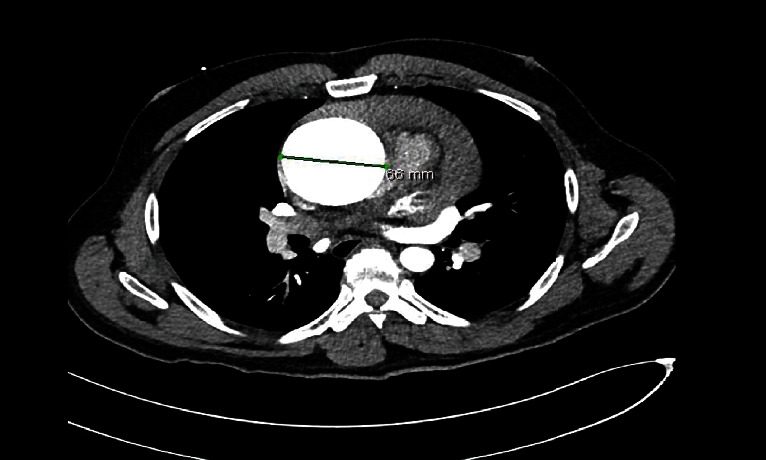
CT imaging with 6.6 cm aneurysm of the ascending thoracic aorta without dissection. Large pericardial effusion.
